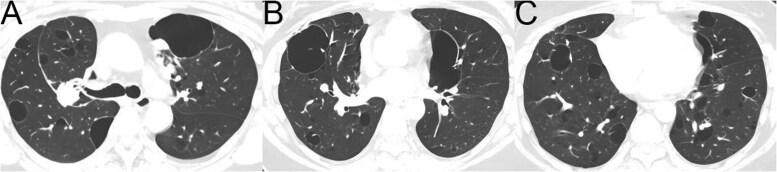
PLCDD in a 46-year-old woman with Sjögren’s disease. CT images (A-C, axial) show multiple bilateral, thin-walled cysts of varying size and shape; intracystic vessels traverse several cyst walls. Abbreviation: PLCDD = pulmonary light chain deposition disease.
